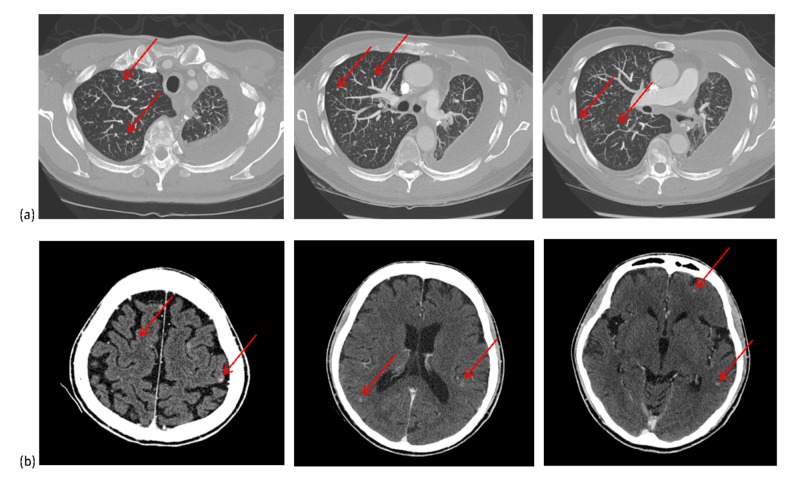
(a) Axial computed tomography (CT) images of the chest and (b) CT images of the brain showing tiny, diffuse, nodular metastases
